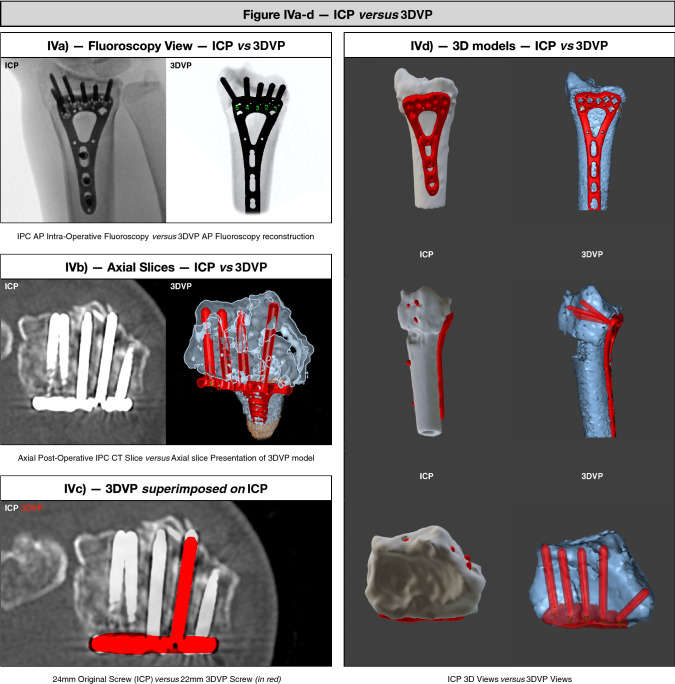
a AP intra-operative fluoroscopy versus AP fluoroscopy of 3DVP model; b axial post-operative CT slice versus axial slice presentation of the 3DVP model; c 24-mm original screw (ICP) versus 22 mm 3DVP Screw (in red); d ICP 3D views versus 3DVP views
